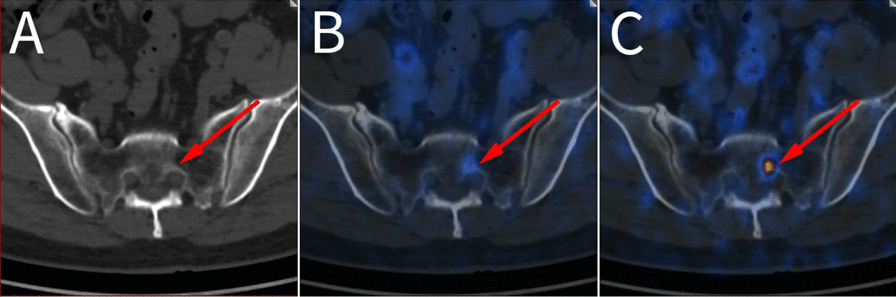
Delayed images on LFOV PET/CT clarified the unclear finding in sacrum in a 73-year-old BCR patient. The sacral metastasis was vaguely visible at the early image (tile B), with a SUV_max_ value of 2.2. Delayed image showed the SUV_max_ value increased to 7.3 (tile C). The CT image showed no clear morphological change (tile A)
